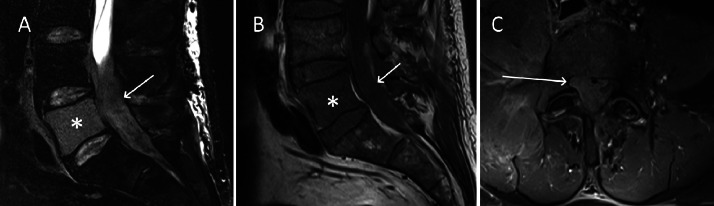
Sagittal T2-weighted image (A) showing a hyperintense L5 vertebra (star) corresponding to leukaemic tumoural infiltration of the bone and a heterogenously hyperintense epidural mass within the spinal canal corresponding to the tumour (arrow). Sagittal T1-weighted image (B) showing a hypointense L5 vertebra (star) when compared to the adjacent muscle, corresponding to tumoural infiltration of the bone. There is a hypointense epidural mass within the spinal canal, extending from L4 to S1 and into the right-sided L5-S1 foramen, corresponding to the tumour (arrow). Axial T1-weighted fat-saturated image acquired after gadolinium contrast administration showing an intensely enhancing mass within the spinal canal, corresponding to the myeloid sarcoma.
